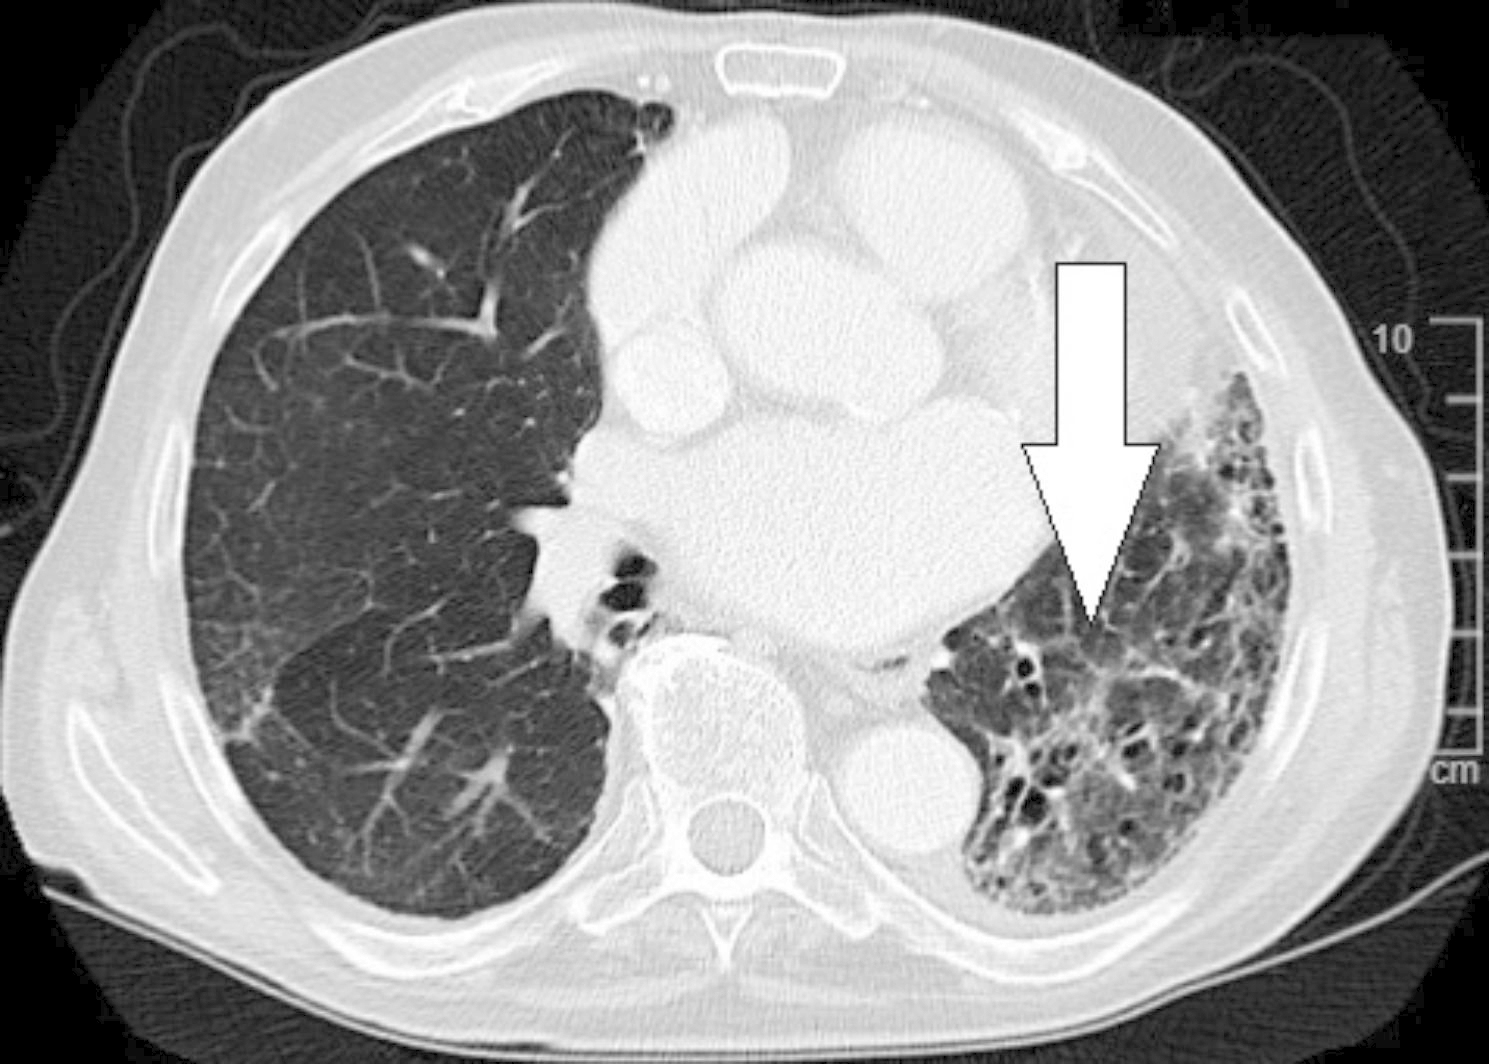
One-year follow-up CT scan excluded short-term tumor recurrence. However, it proved unilaterally pronounced aggravation of fibrotic lung disease in the left lower lobe (arrow)
